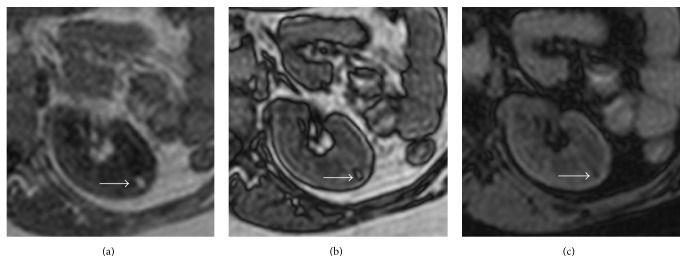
Small left kidney typical AML. Axial GE T1-weighted IP image (a); axial GE T1-weighted OP image (b); axial GE T1-weighted fat-sat image. A small renal AML with typical appearance (arrow): high signal intensity on T1-weighted IP image, “India-ink” artifact on T1-weighted OP image, and loss of signal intensity on T1-weighted fat-sat image.
